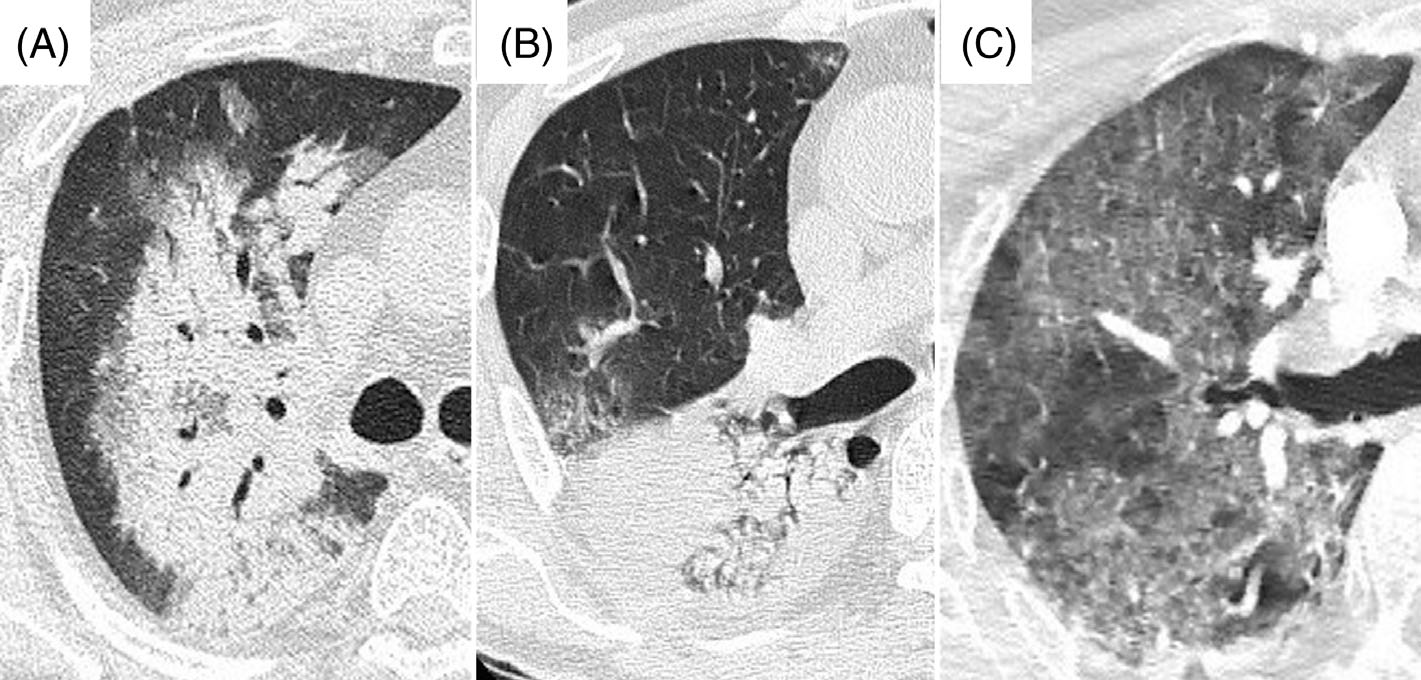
Outline of the study. ^*^All patients with respiratory failure suspected of ARDS underwent chest CT, and those suspected of infectious pneumonia on CT were excluded. One hundred and twelve patients were ineligible because of chronic interstitial lung diseases, two patients recovered from cardiopulmonary arrest, and one had pneumothorax and another one had advanced neoplasms and was expected to live for less than 2 months. ARDS, acute respiratory distress syndrome; CT, computed tomography
